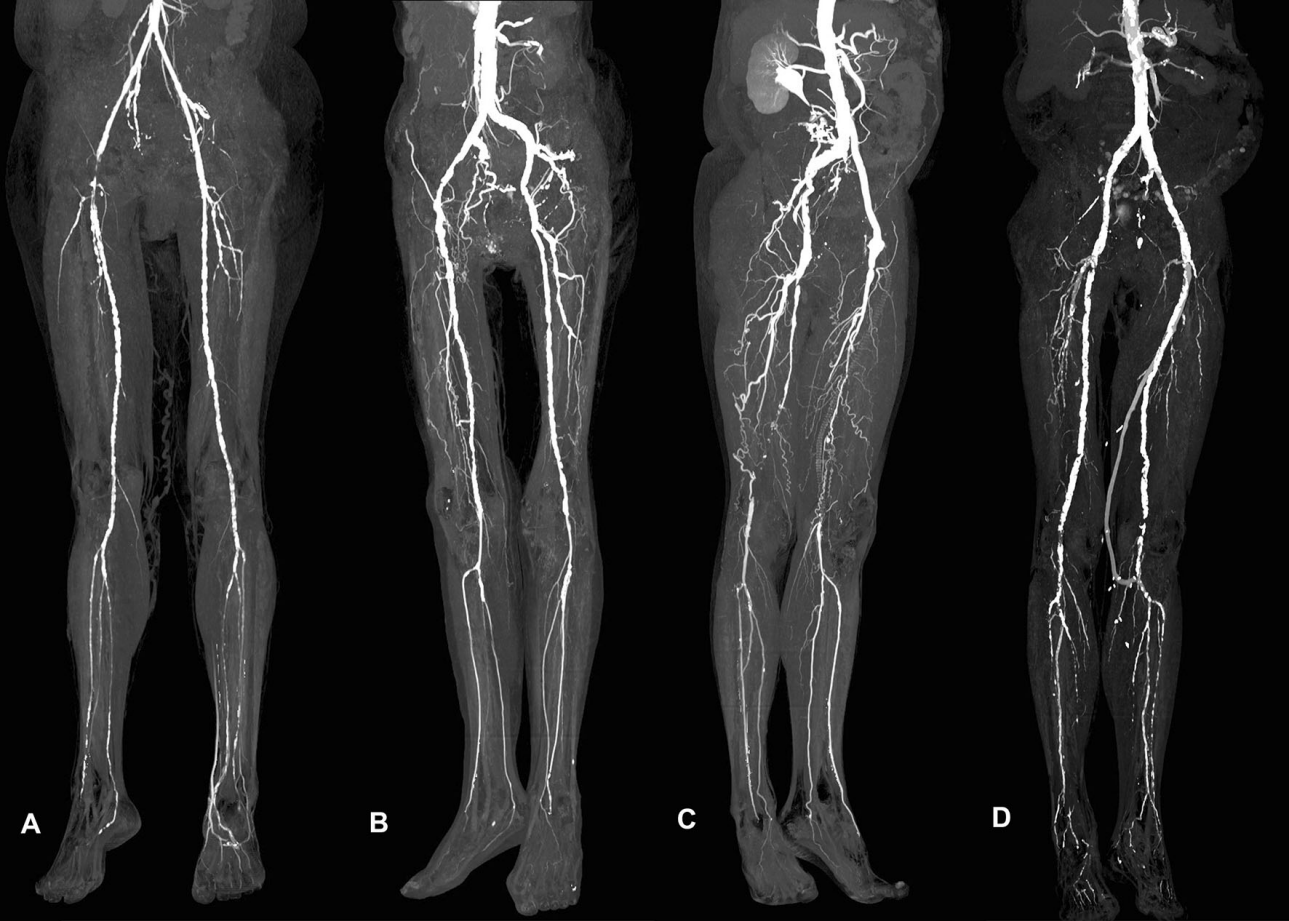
Maximum intensity projections (MIP) of the lower limb CTA after bone subtraction. A Right SFA occlusion in 46-year-old female causing Rutherford I symptoms. B Significant stenosis of right CIA in 57-year-old female patient with metastatic cervical carcinoma, referred for CTA with suspected thromb-embolic source in the peripheral arteries. Multiple vascular caliber changes of CIA and SFA are visible bilaterally. C 64-year-old male patient with left CFA aneurysm (6.8 × 4.6 cm), occluded left femoro-popliteal bypass and bilateral SFA occlusion including proximal PA (level P1). D Rutherford III complaints in 66-year-old male patient caused by stenosis of left femoro-popliteal bypass at the level of distal anastomosis and complete right SFA occlusion. *CFA* common femoral artery, *CIA* common iliac artery, *CTA* computed tomographic angiography, *SFA* superficial femoral artery, *EIA* external iliac artery, *PA* popliteal artery
